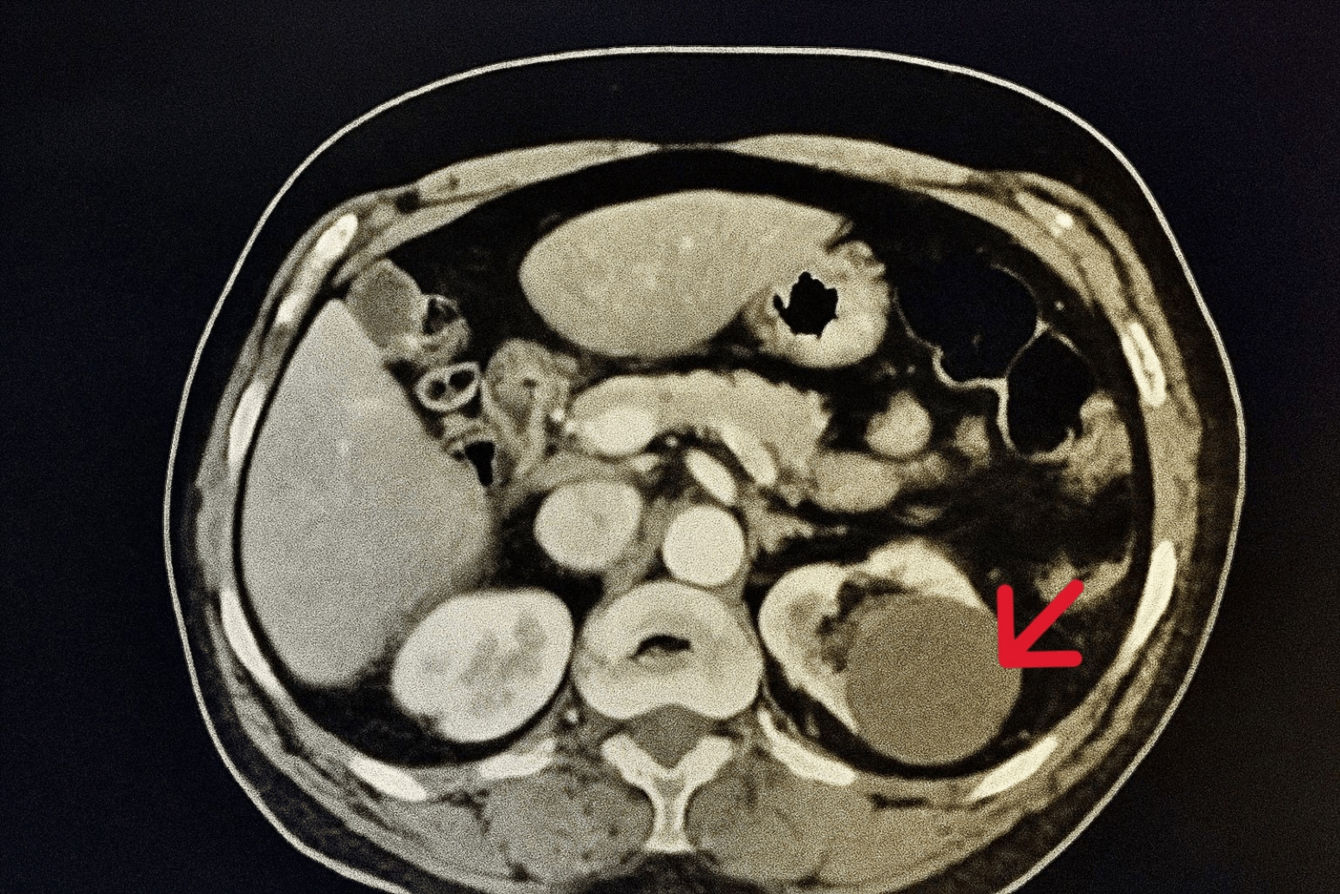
CT-Scan Abdomen Showing Left Renal Mass (RCC) The red arrow indicates a renal mass suggestive of renal cell carcinoma (RCC).
